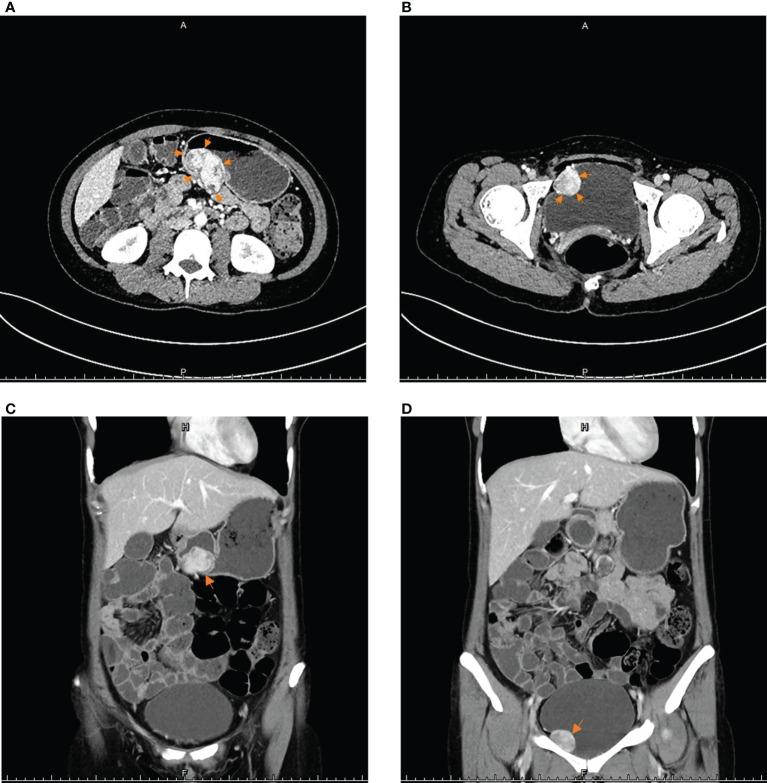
The imaging results of contrast-enhanced CT scans of the abdomen and pelvis: (A, C) The arrows point to a soft tissue mass approximately 33 mm×54 mm in the posterior wall of the gastric body. (B, D) The arrows point to a protrusion into the right wall of bladder, approximately 26 mm×29 mm in size of round lumps.
